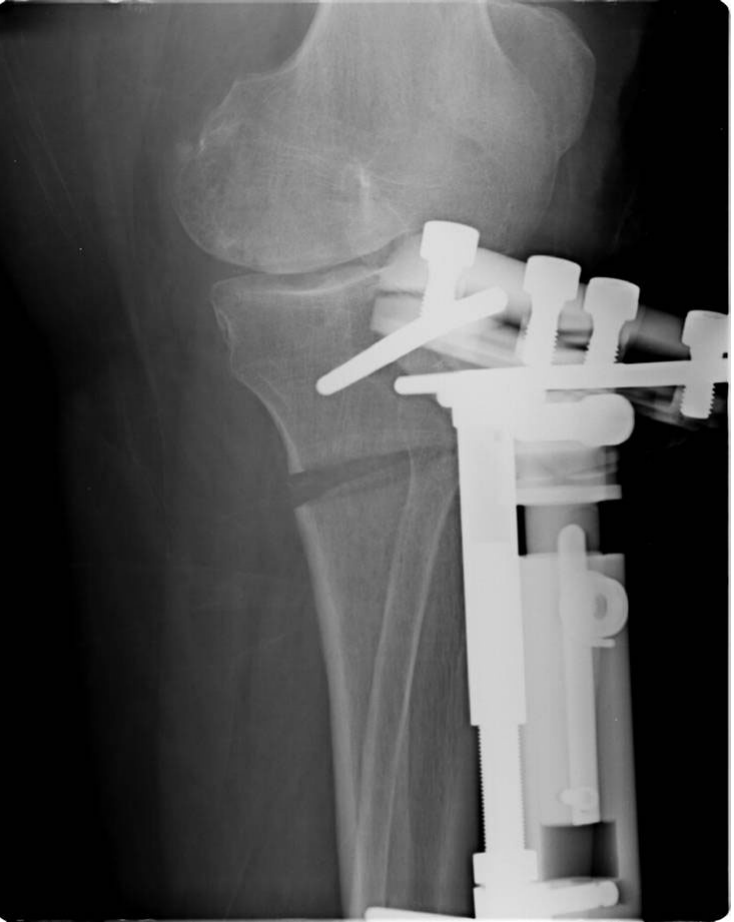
Radiograph of high tibial osteotomy using the hemicallotasis technique.
